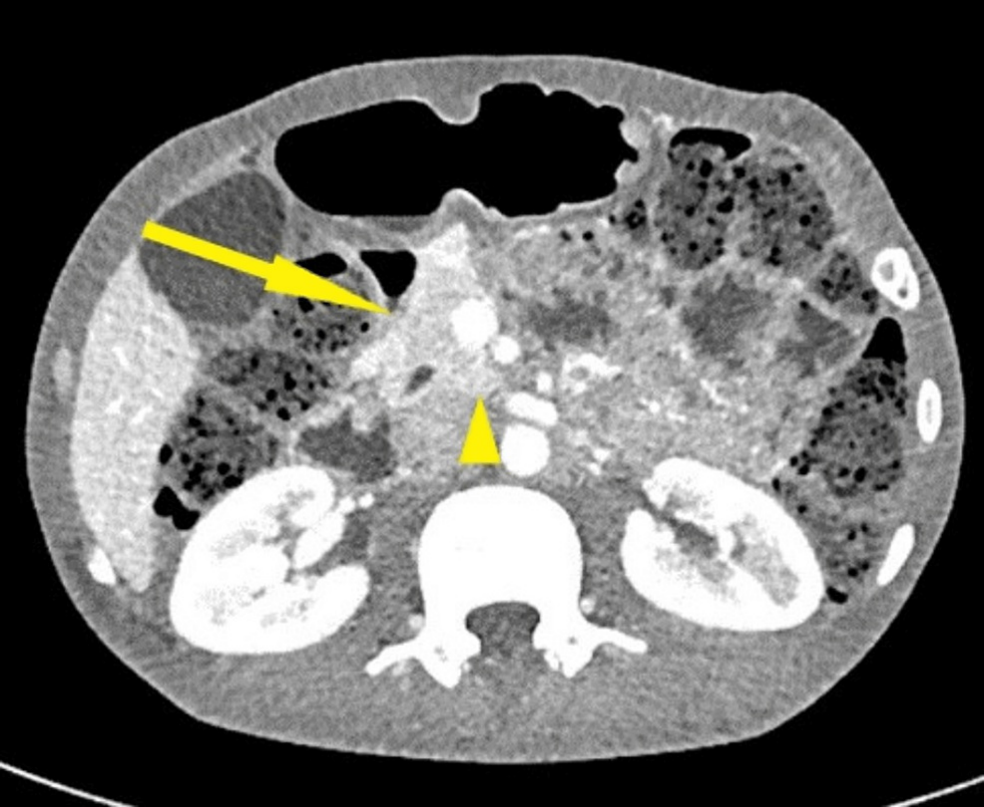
CECT showing the head (solid arrow) and uncinate process (arrowhead) of the pancreas CECT: contrast-enhanced computed tomography
